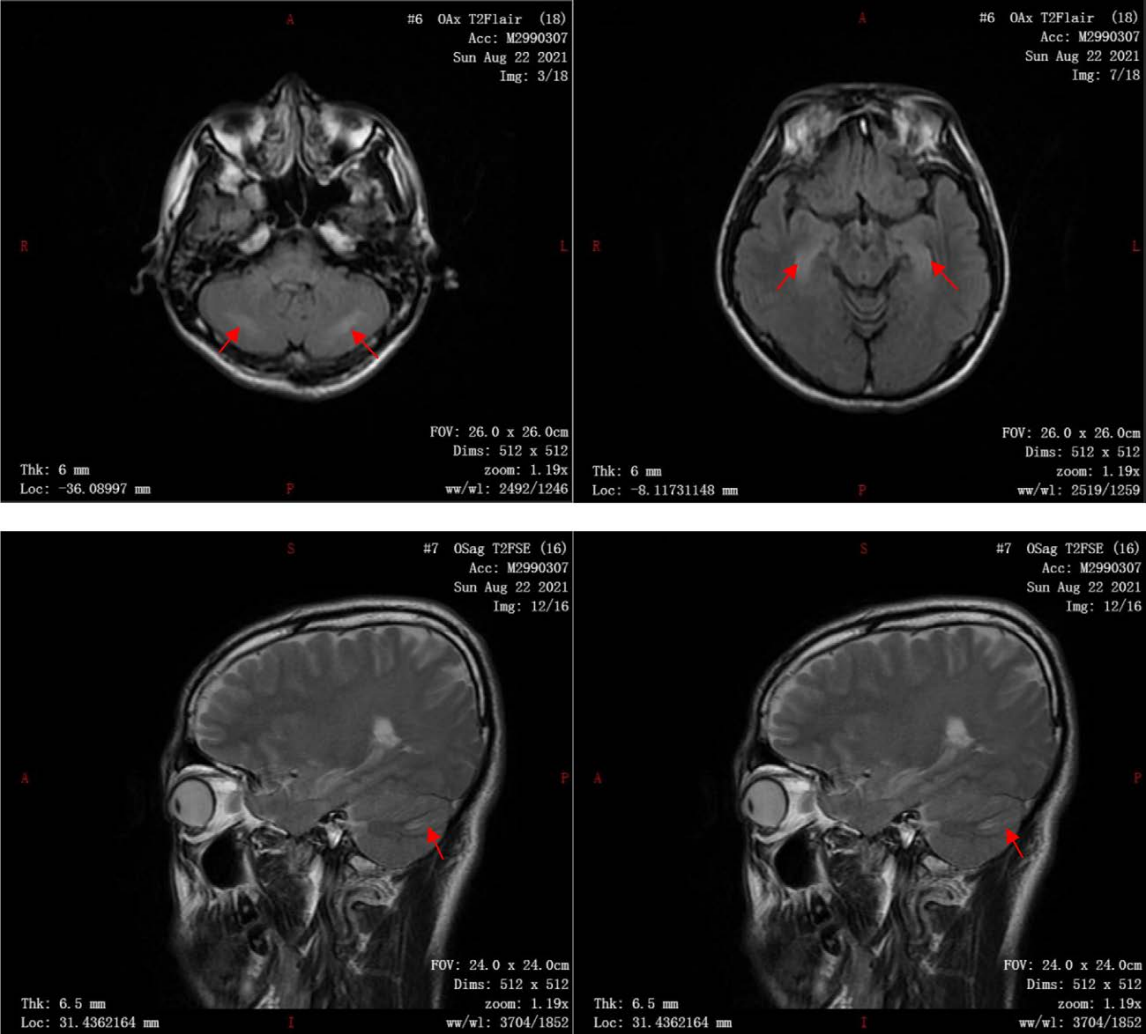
Patches of slightly long T2 signal with asymmetrical distribution in the bilateral cerebellar hemispheres and bilateral hippocampal regions were seen, with slightly high signal in the pressure water and diffusion-weighted imaging (DWI) sequences.
